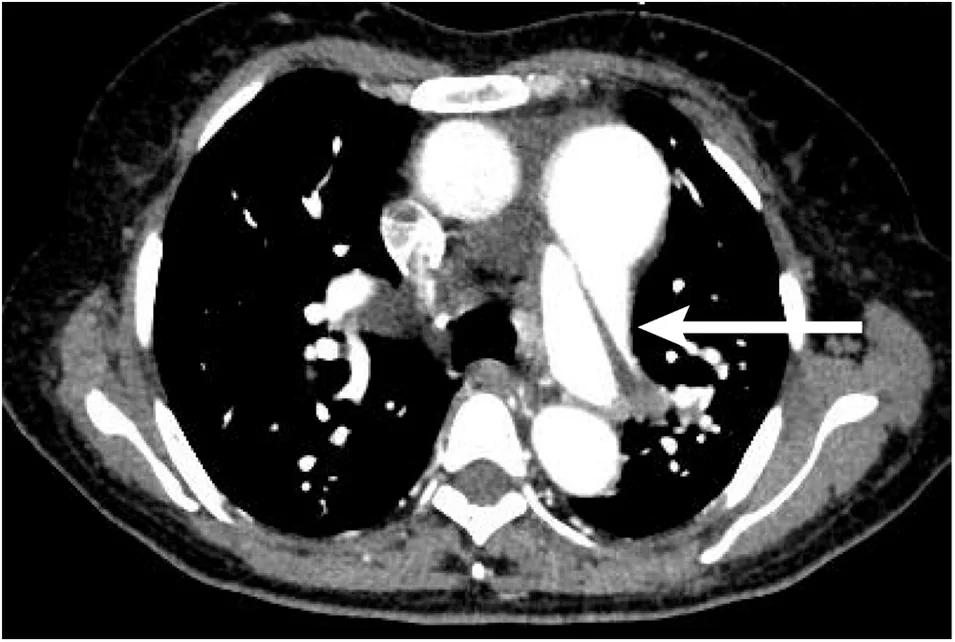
Enhanced CT revealed the left-side pulmonary artery dissection located at the beginning of the left pulmonary artery (arrow).
